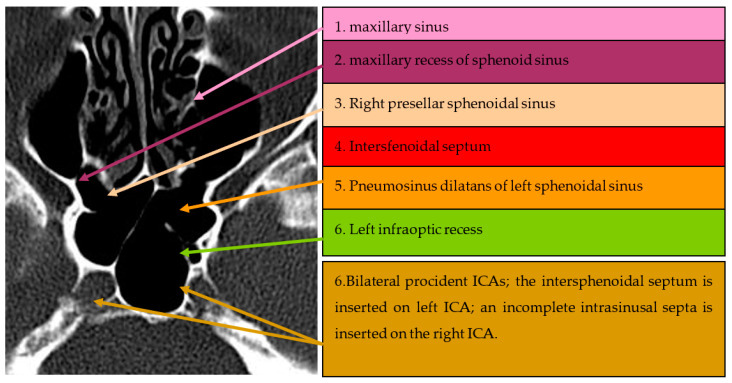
Axial CT section of paranasal sinuses with procidence of both ICAs, on which are inserted two septa.
